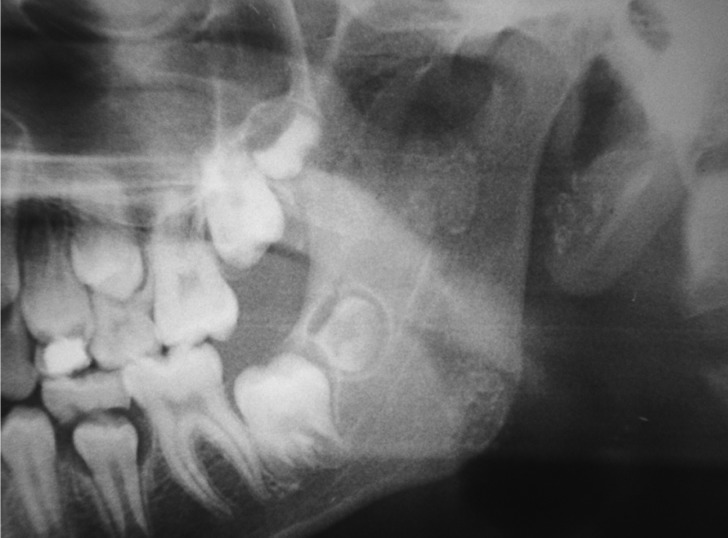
Panoramic Radiography. Notice the multiple radiopaque masses throughout the soft tissue in the parotid region.
